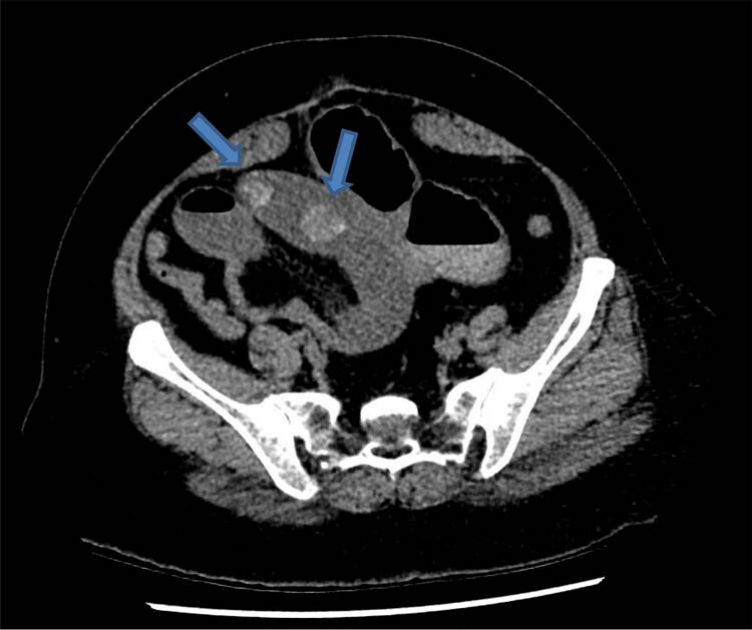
Axial CT image demonstrates two 25-mm gallstones (blue arrows) in a loop of small bowel in the right lower quadrant.
